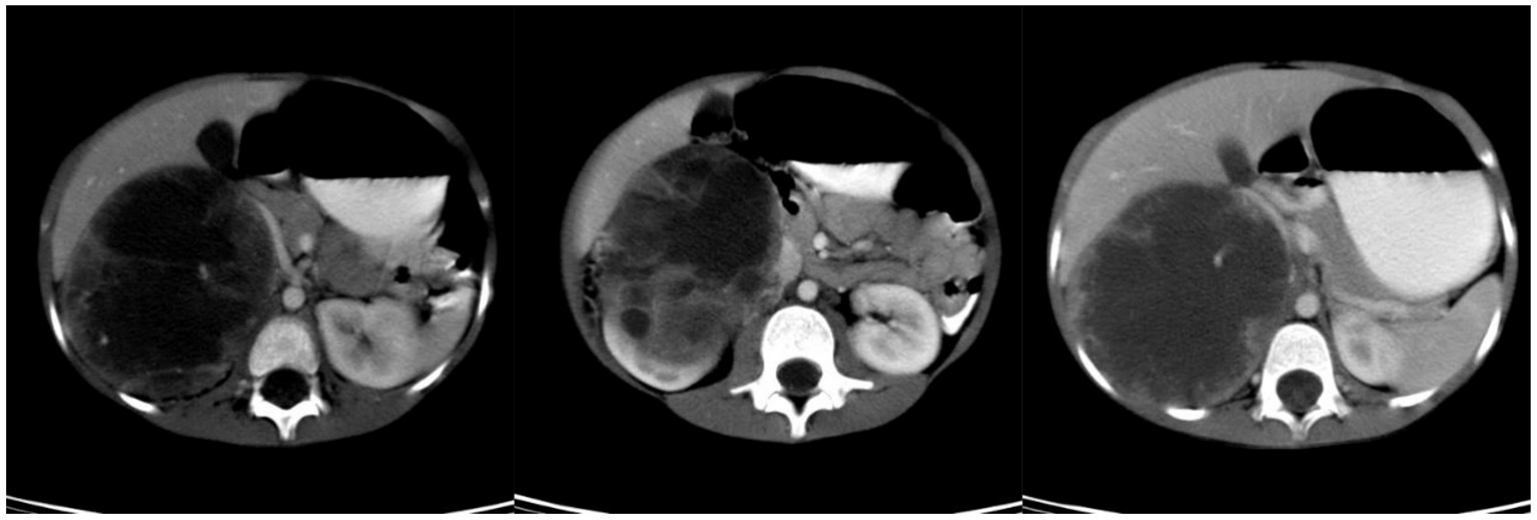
Preoperative CT scan of patient E.
